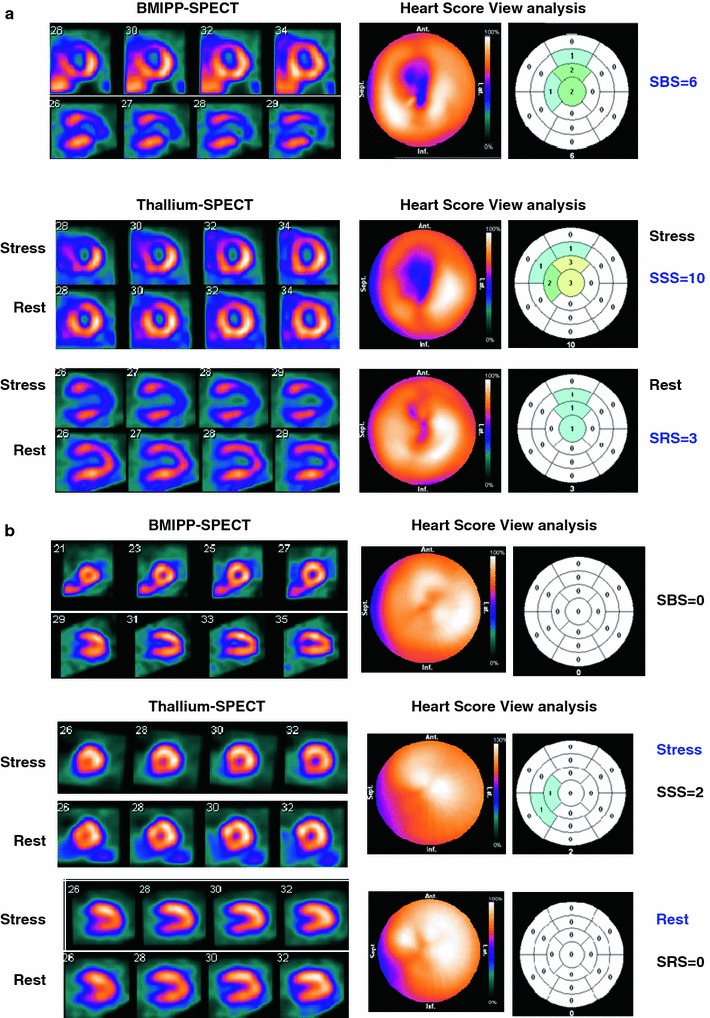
SPECT, polar and heart score view maps of stress and rest thallium myocardial perfusion and rest BMIPP images. a Suspected stable coronary artery disease in a 77-year-old hypertensive, diabetic woman. Automated scoring system scored 6, 10 and 3 for BMIPP, stress and rest thallium, respectively, indicating intermediate risk for coronary events. Ninety days later, she underwent emergency percutaneous coronary intervention to treat acute coronary syndrome. b Suspected coronary artery disease in a 61-year-old hypertensive, dyslipidemic man with a smoking history. Automated scoring system scored 0, 2 and 0 for BMIPP, stress and rest thallium images, respectively, indicating low risk for cardiac events. He has remained free of cardiac events for 4 years
